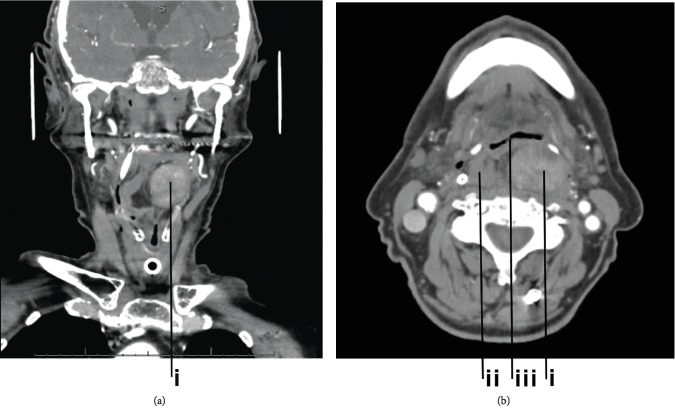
CT angiogram of neck ((a) coronal, (b) axial) demonstrating a 3.5 cm heterogeneous lesion in the posterior wall of the hypopharynx in a submucosal location (i) and a smaller 1.7 cm lesion of the right posterior wall of the hypopharynx (ii). The mass caused severe narrowing of the air column at the hypopharynx (iii).
